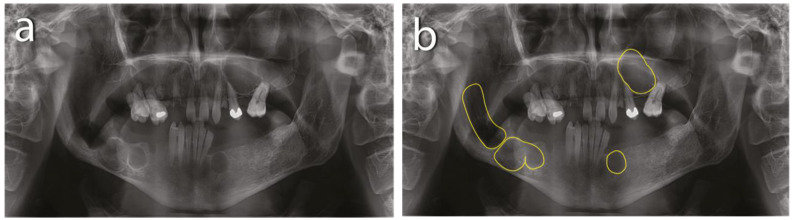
Preoperative X-rays: (a) residual roots and multiple keratocysts are visible on the orthopantomogram; (b) keratocysts are marked with yellow lines.
